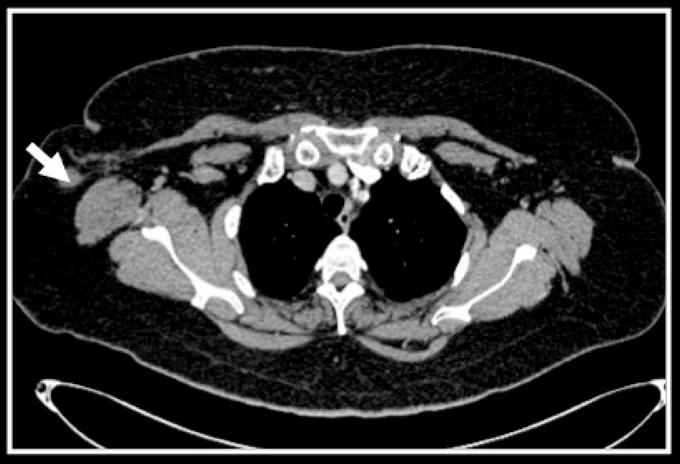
Contrasted CT thorax in axial section showing a well-defined soft tissue density mass measuring 1.7 cm seen in the right axillary region (white arrow).
